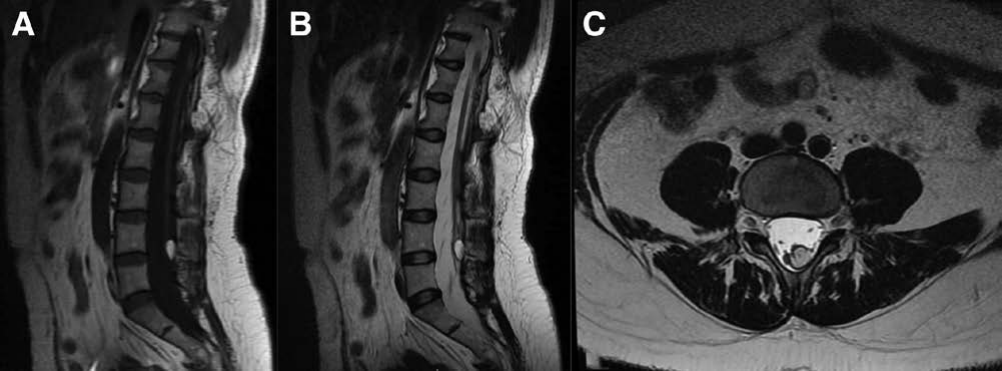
(A) The preoperative lumbar spine plain MRI scan using a T1 sequence in the sagittal plane. (B) The preoperative lumbar spine plain MRI scan using a T2 sequence in the sagittal plane. (C) The preoperative lumbar spine plane MRI scan using a T2 sequence in cross-section. MRI = magnetic resonance imaging.
